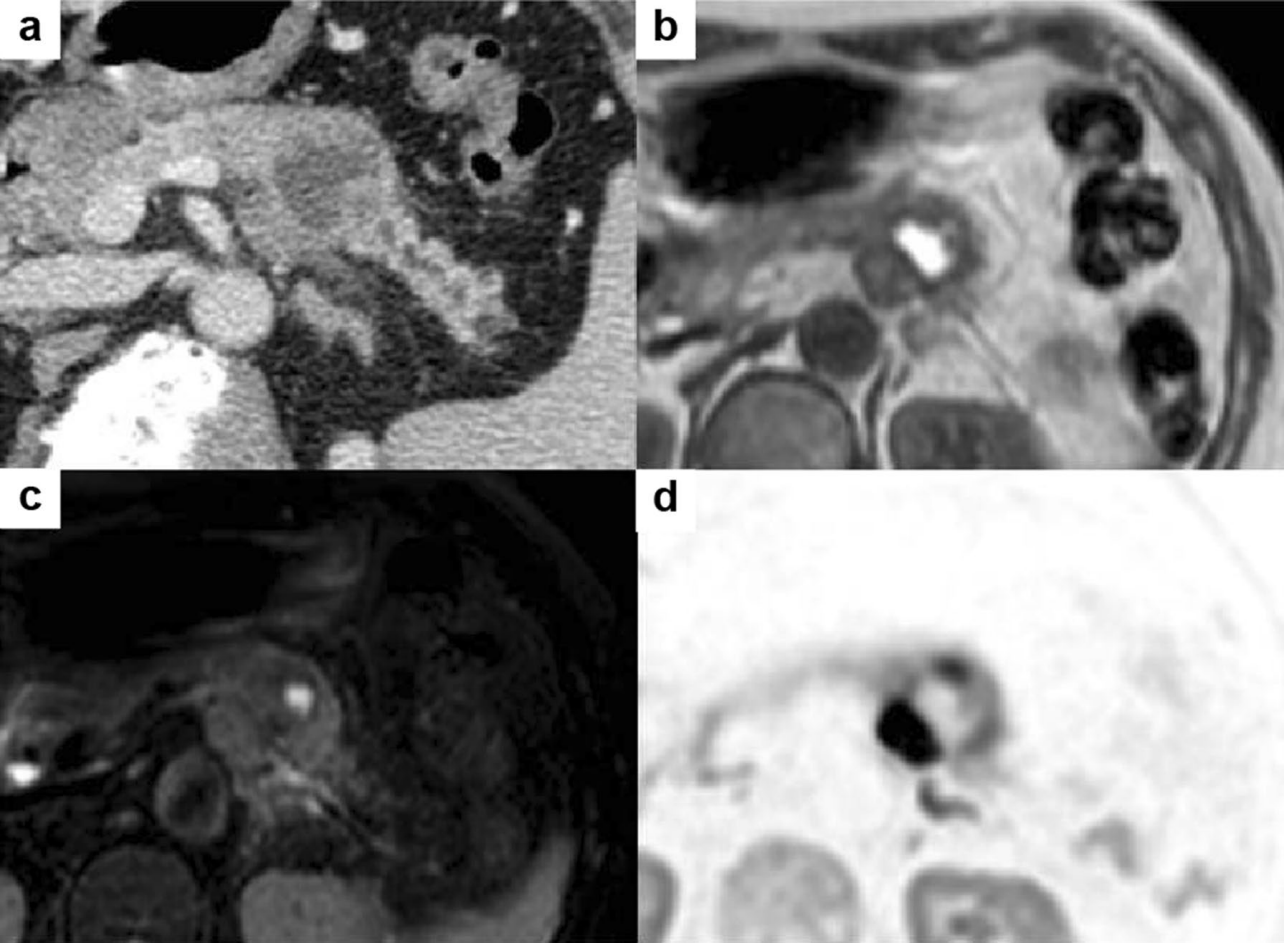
Computed tomography image. a Mass in the tail of the pancreas with pancreatic ductal dilation. The central mass is hyperintense on T1-weighted image (b) and hypointense on T2-weighted image (c). d Peripheral rim of the mass is hyperintense on diffusion-weighted image
